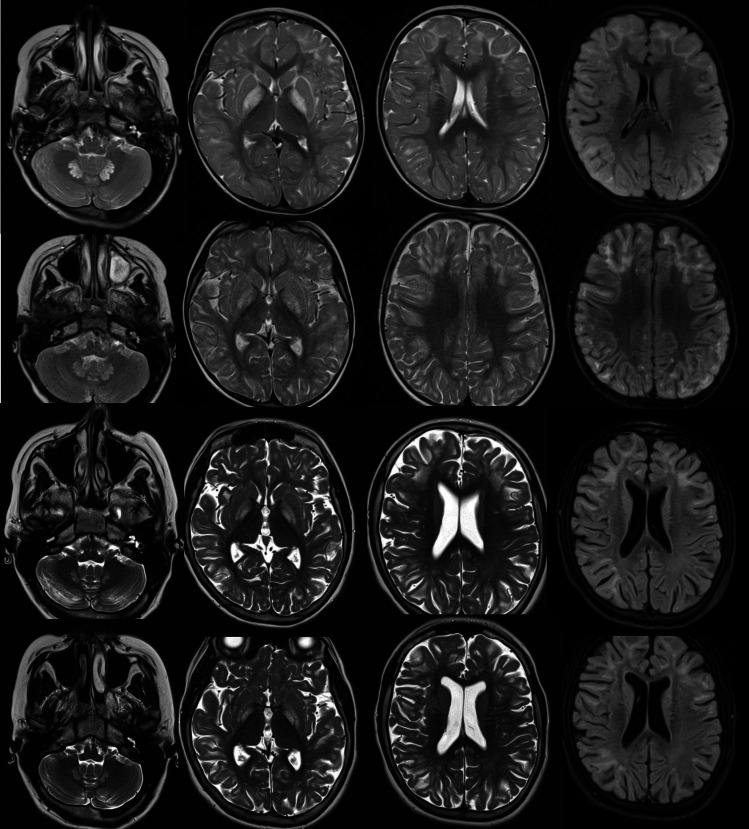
Brain MR examinations, T2 W, and FLAIR images
